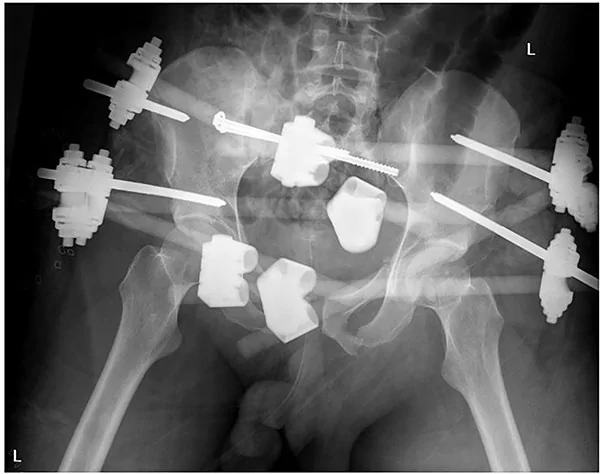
Pelvic radiograph showing stabilized pelvic fracture with an external fixator and supracetabular pins, in addition to sacroiliac screws.
